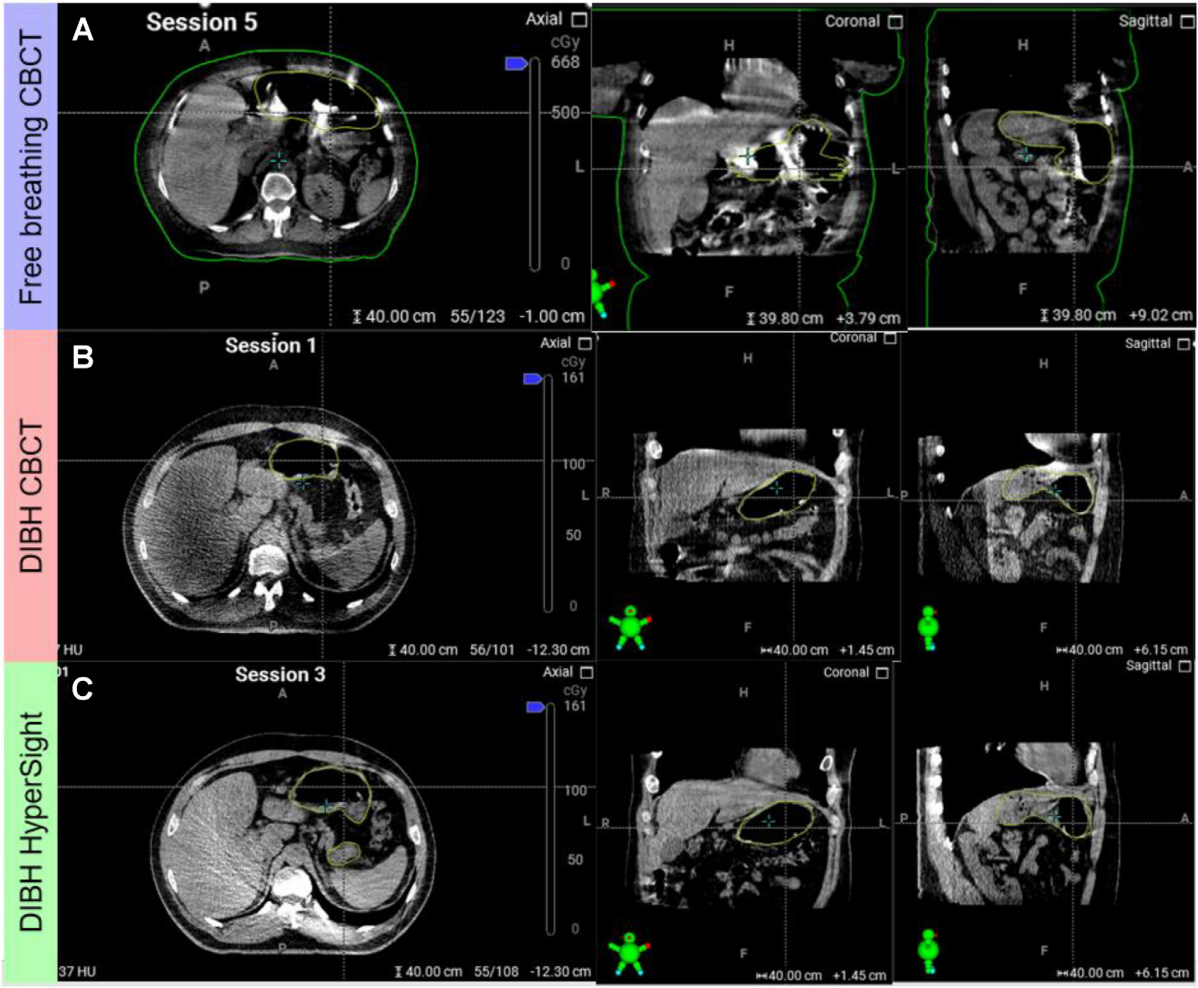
Example cone-beam computed tomography (CBCT) image quality for a free-breathing abdomen patient (a) and another patient with mucosa-associated lymphoid tissue lymphoma treated with conventional CBCT (b) and HyperSight (c) with surface guided radiation therapy deep inspiration breath-hold (DIBH). The green contour represents the stomach (clinical target volume) contour.
